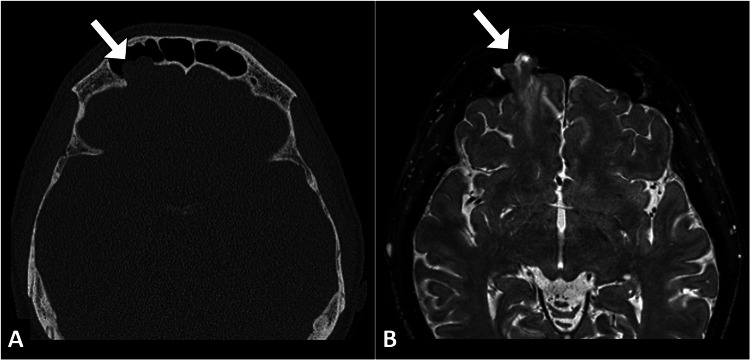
Axial CT head image with a bone window (A) revealing a notable bone defect (arrow) in the right anterior cranial fossa. The corresponding T2-weighted MR image (B) demonstrates herniation of brain tissue through this defect (arrow), consistent with a frontal meningoencephalocele. CT: computed tomography; MR: magnetic resonance
